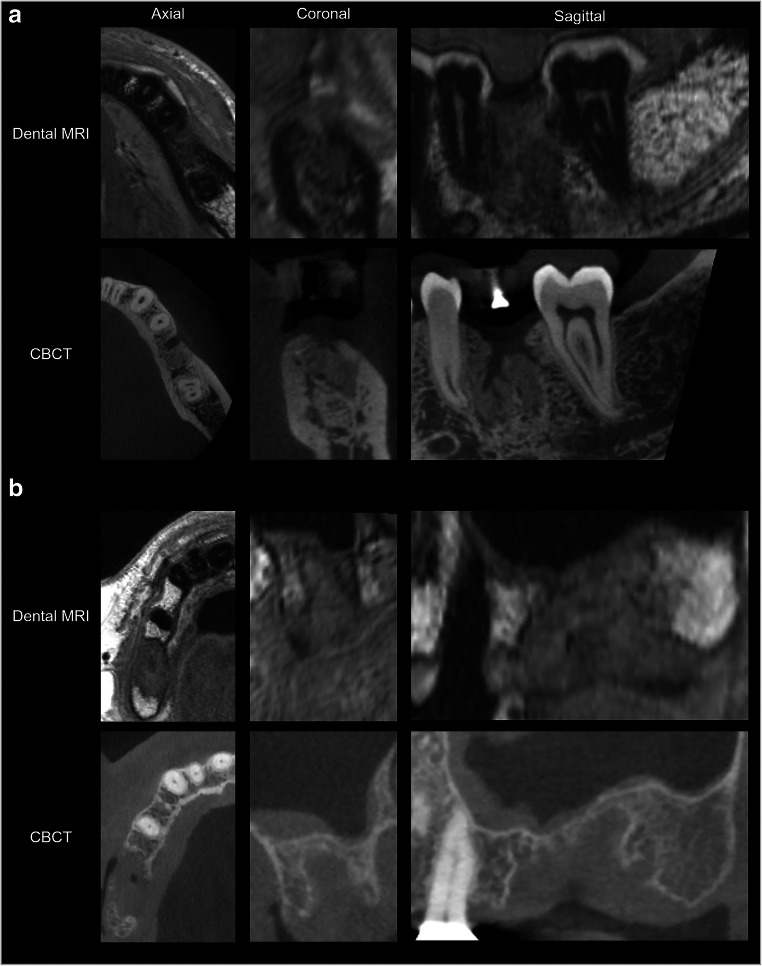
Two cases of extensive, bone loss in dental MRI and CBCT. Based on dental MRI, case a was misclassified as sufficient bone without the need for bone augmentation. At implant site 36, the preserved outer shape of the alveolar bone was misleading. In the second case (b), dentists correctly identified extensive bone loss in implant site 17 from the dental MRI examination. It was confirmed by CBCT that simultaneous implant insertion and bone augmentation were therefore not possible
